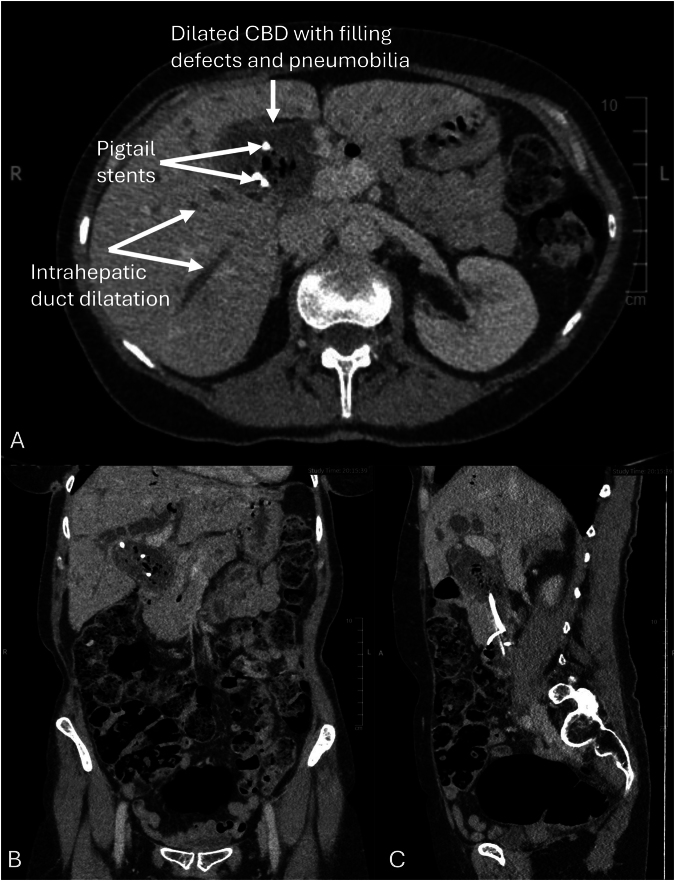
Axial (A), coronal (B) and sagittal (C) computed tomography images showing dilated intra- and extrahepatic bile ducts, pneumobilia, and multiple filling defects in the common bile duct (CBD) next to the pigtail stents.
